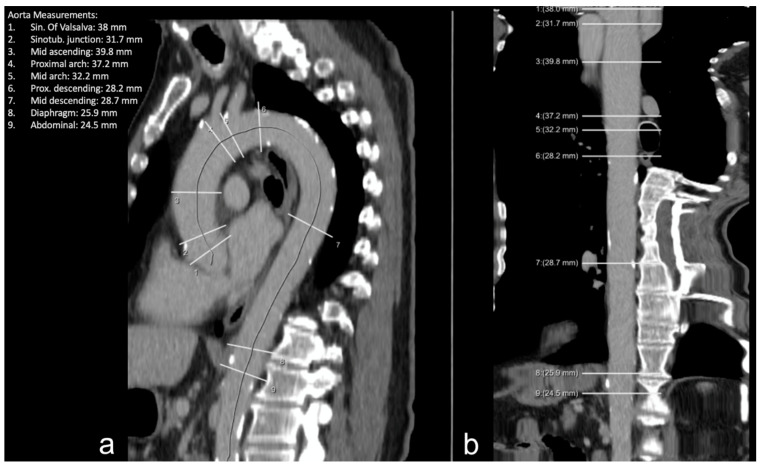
Example of the thoracic aorta imaged via CT in plane reformatted along the center line (a) and straightened reconstruction (b). White lines: measurement planes of the diameter at each dedicated location.
